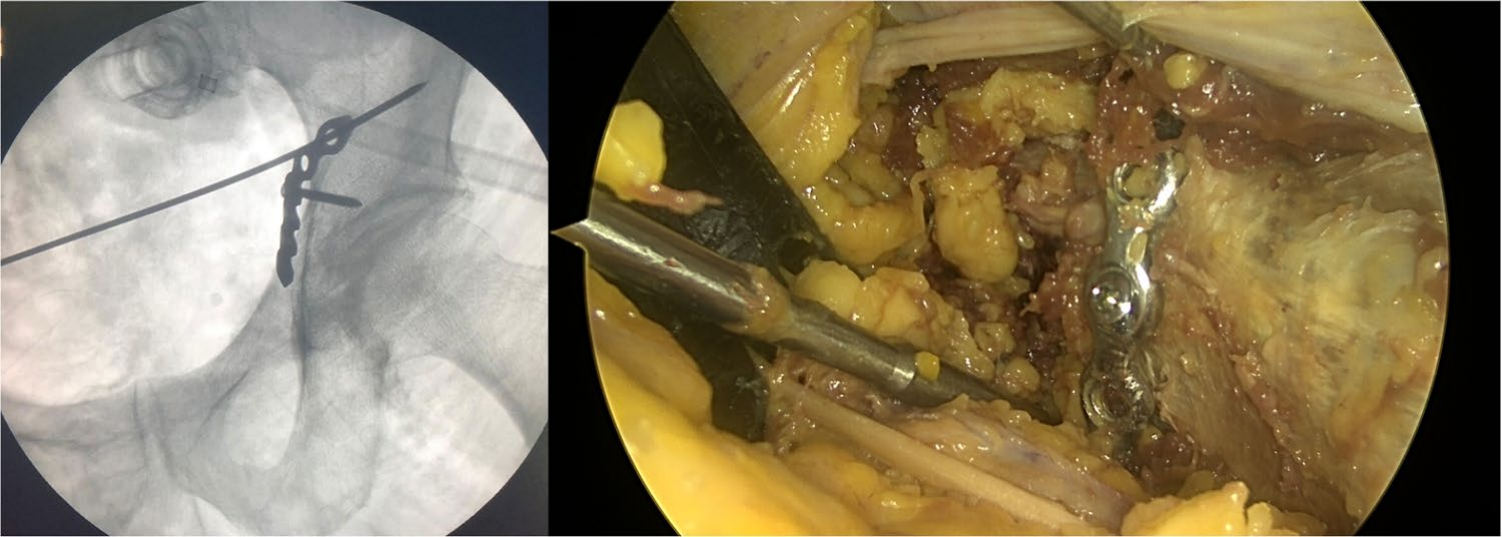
Shows an example of a plate positioned to the posterior column with a corresponding radiograph. The screwdriver is pointing into the greater sciatic notch indicating the posterior border of the posterior column
